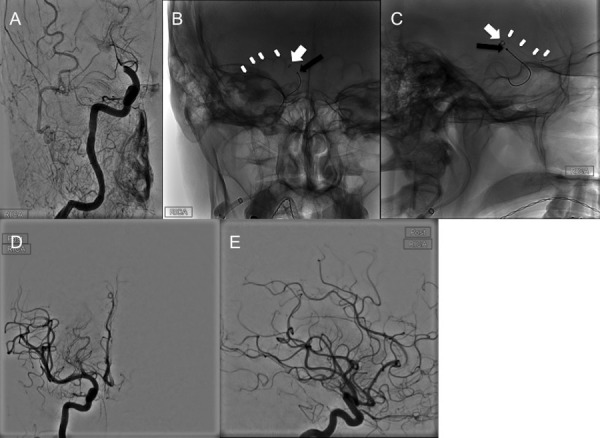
Case 2 (A) Frontal DSA run of the right anterior circulation demonstrates abrupt cutoff of the supraclinoid right ICA just distal to the right posterior communicating and right anterior choroidal arteries (long white arrow). The tip of the BGC is seen at the cervical ICA (short white arrow); this was soon repositioned slightly more proximal. (B) Frontal and (C) lateral magnified radiographs of the head demonstrating the triaxial assembly for mechanical thrombectomy for the second pass as follows: The inflated BGC (short black arrow) in the right cervical ICA, the distal marker of the intermediate Arc™ catheter facing the proximal aspect of the occluded right M1 segment (short white arrows), and the stent retriever which has been deployed from a proximal M2 branch down to the proximal M1 (white arrowheads). Note the microcatheter used to deliver the stent-retriever is inside the intermediate catheter (long black arrows). Prior to the thrombectomy pull the microcatheter was removed (ARTS technique). (D) and (E): Final DSA runs of the head with frontal and lateral views demonstrate a TICI 3 outcome. RICA = Right internal carotid artery.
